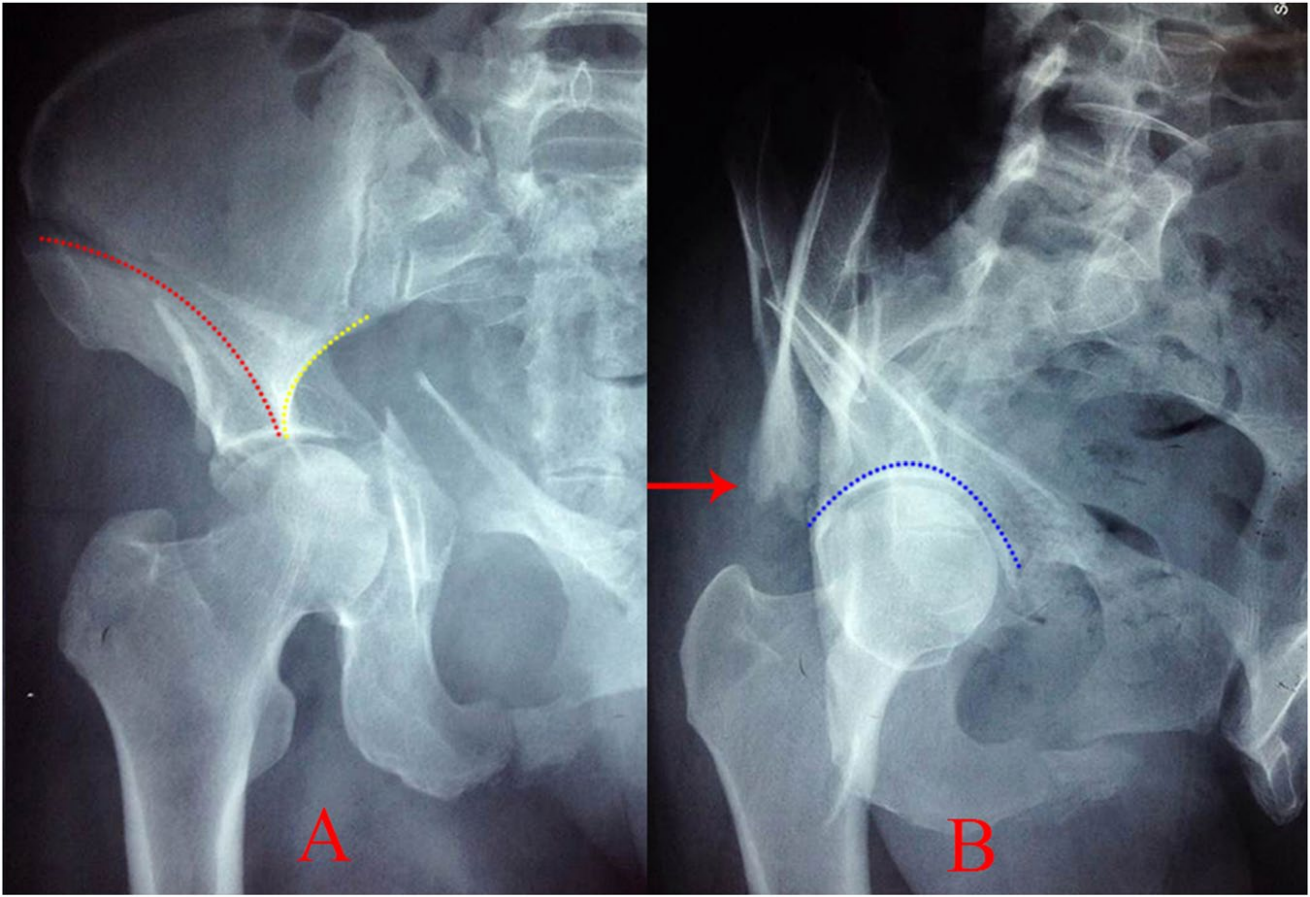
The radiographic characteristics of both-column fractures. (A) Iliac wing fracture line (red dotted line) and curved appearance (yellow dotted line), (B) the false congruence (blue dotted line) and the spur sign (arrow) are apparent.
